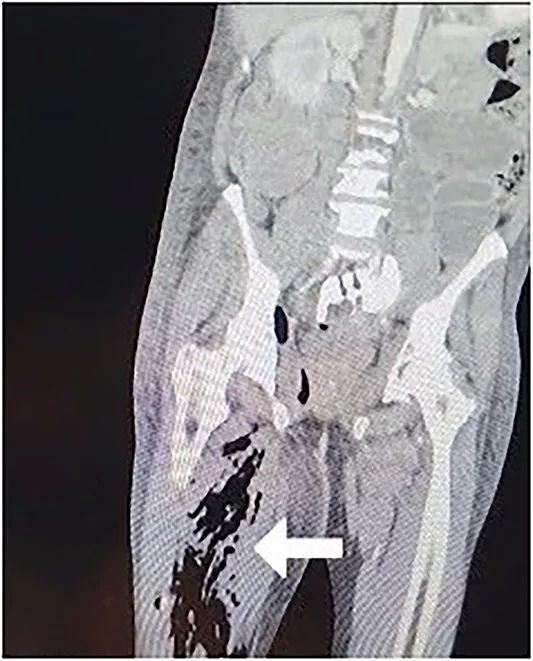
Coronal view showing extension of ischiorectal fistula into thigh compartments as seen on CT pelvis and lower extremity. Arrow: intramuscular gas and fluid tracking along the fascial planes.
